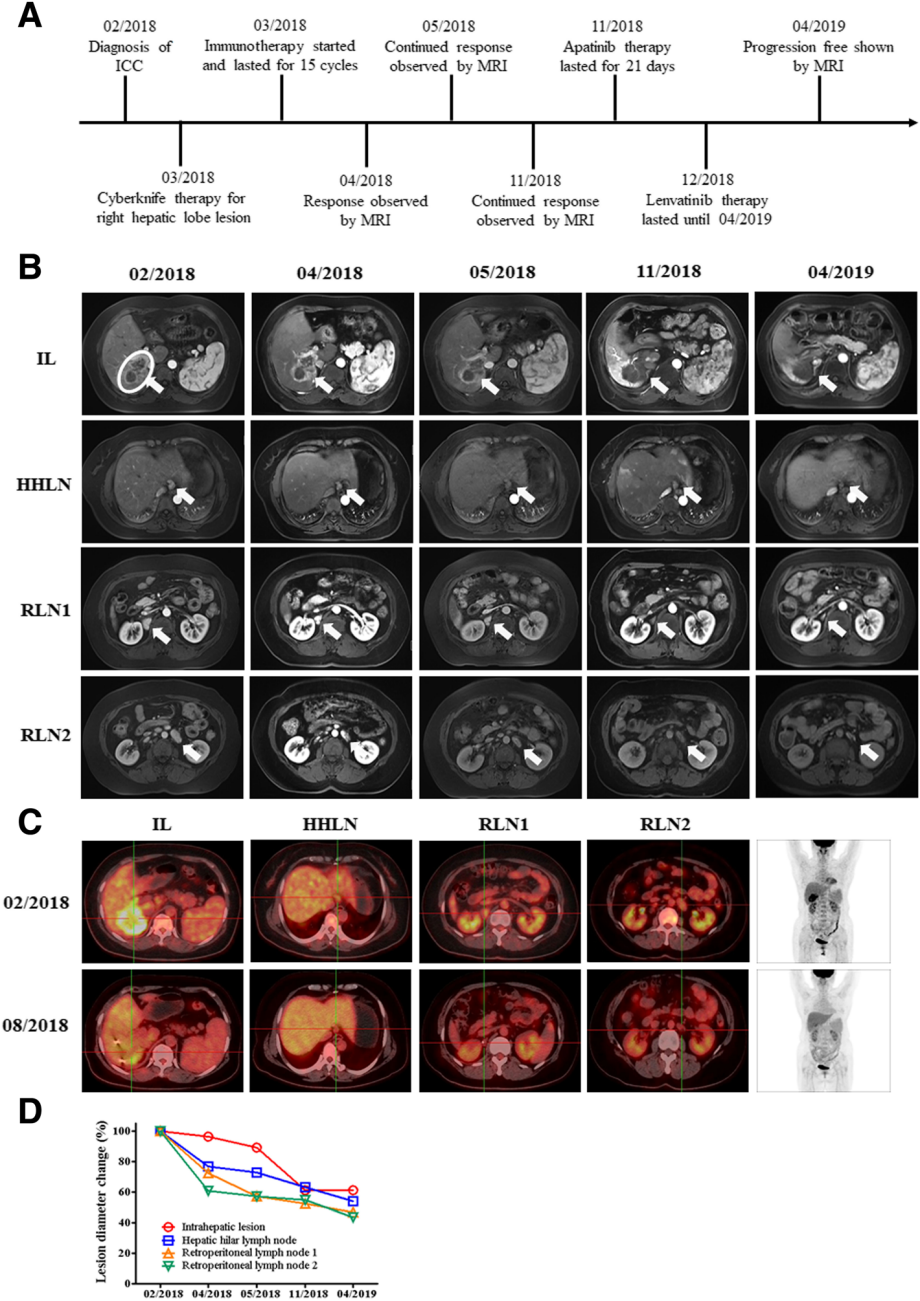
Images describing the condition and therapeutic responses of patient A. a scheme shows the time course of patient A in diagnosis the therapy. b MRI images show the therapeutic response of patients following a series of treatment. The circle indicates the target lesion/region for radiotherapy, and arrows in figures indicate the position of lesions. c PET-CT images show the therapeutic response of patients following a series of treatment. d Statistics of lesion diameter reduction for all lesions of patient A. IL: Intrahepatic lesion, HHLN: Hepatic hilar lymph node, RLN1: Retroperitoneal lymph node 1, RLN2: Retroperitoneal lymph node 2
